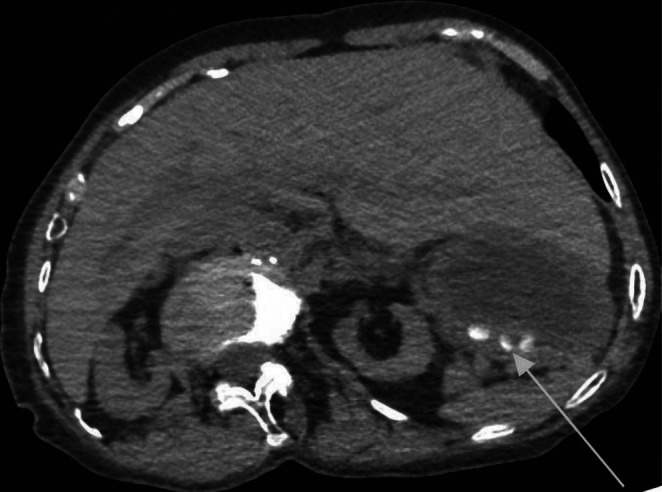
Axial CT scan of the abdomen in Case 2 showing multiple hyperdense structures in the stomach (arrow), consistent with retained drug fragments. The finding supported the decision to perform catharsis.
